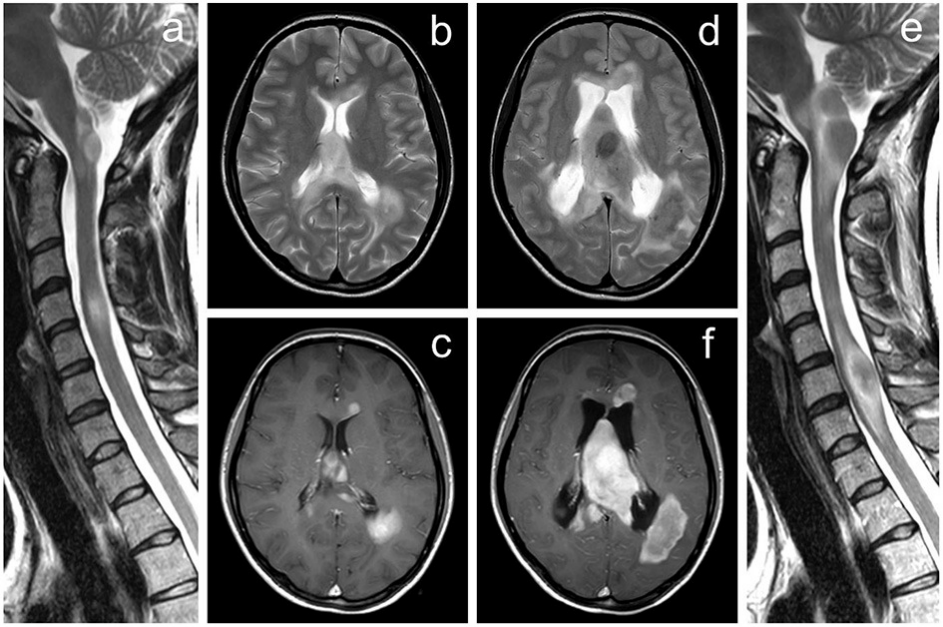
MRI of the brain and cervical spine documenting a significant progression of the tumefactive lesions. (a–c) Examination performed ~5 years after the initial diagnosis of multiple sclerosis, (d–f) follow-up examination after further 22 months. Several tumor-like enhancing lesions located around the lateral ventricles are visible on axial T2-weighted images (b,d) and contrast-enhanced axial T1-weighted images (c,f). Extensive mass lesion located within the septum pellucidum finally caused the hydrocephalus by obstruction of the interventricular foramina; a significant dilatation of the lateral ventricles is seen on follow-up examination (d,f). The progressive course of the disease is also demonstrated by T2-weighted images of the cervical spine in the sagittal plane (a,e).
